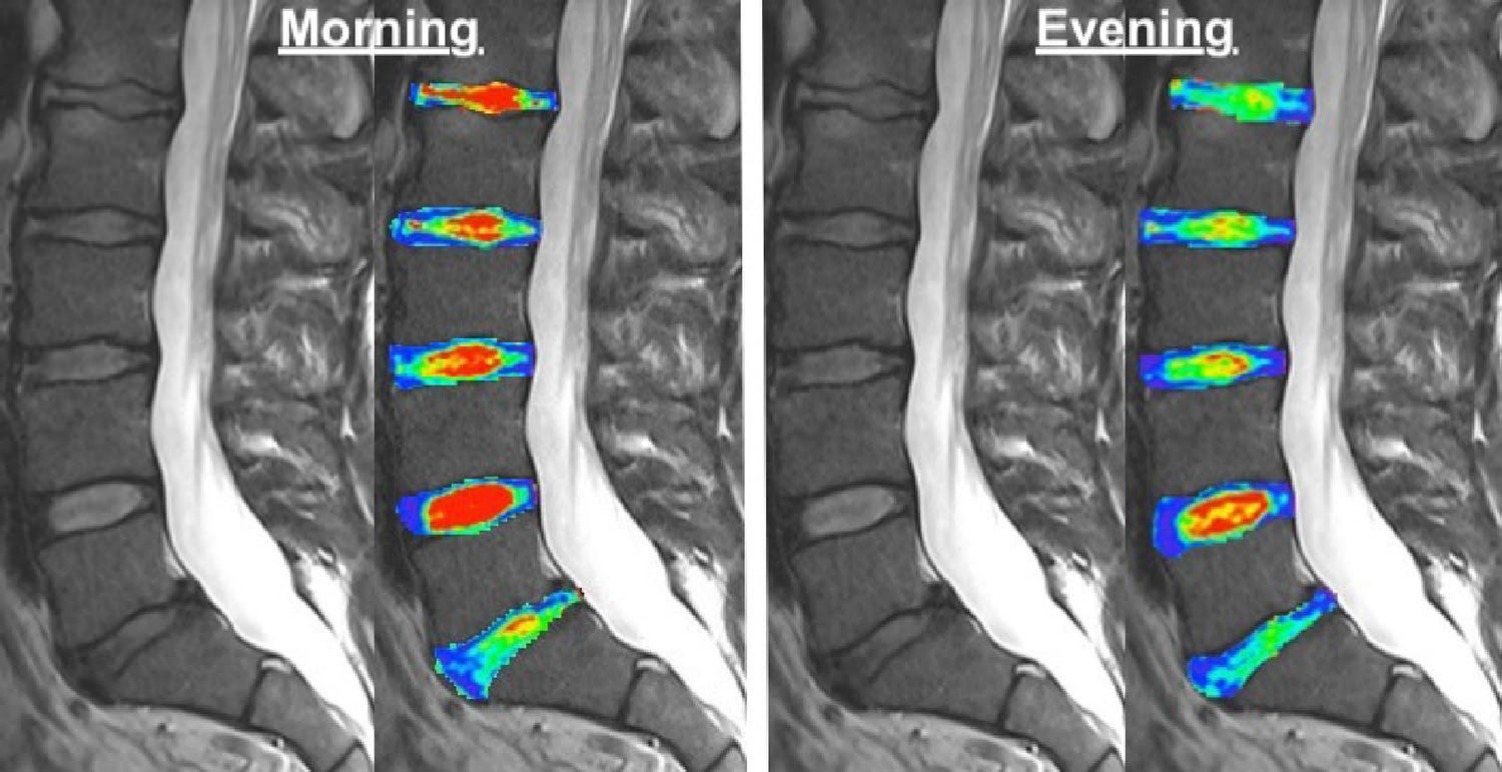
Intervertebral discs (IVDs). These sagittal magnetic resonance images (MRI) of a 53 year old male display higher T2-values of IVDs in the morning (red) than in the evening (green).
